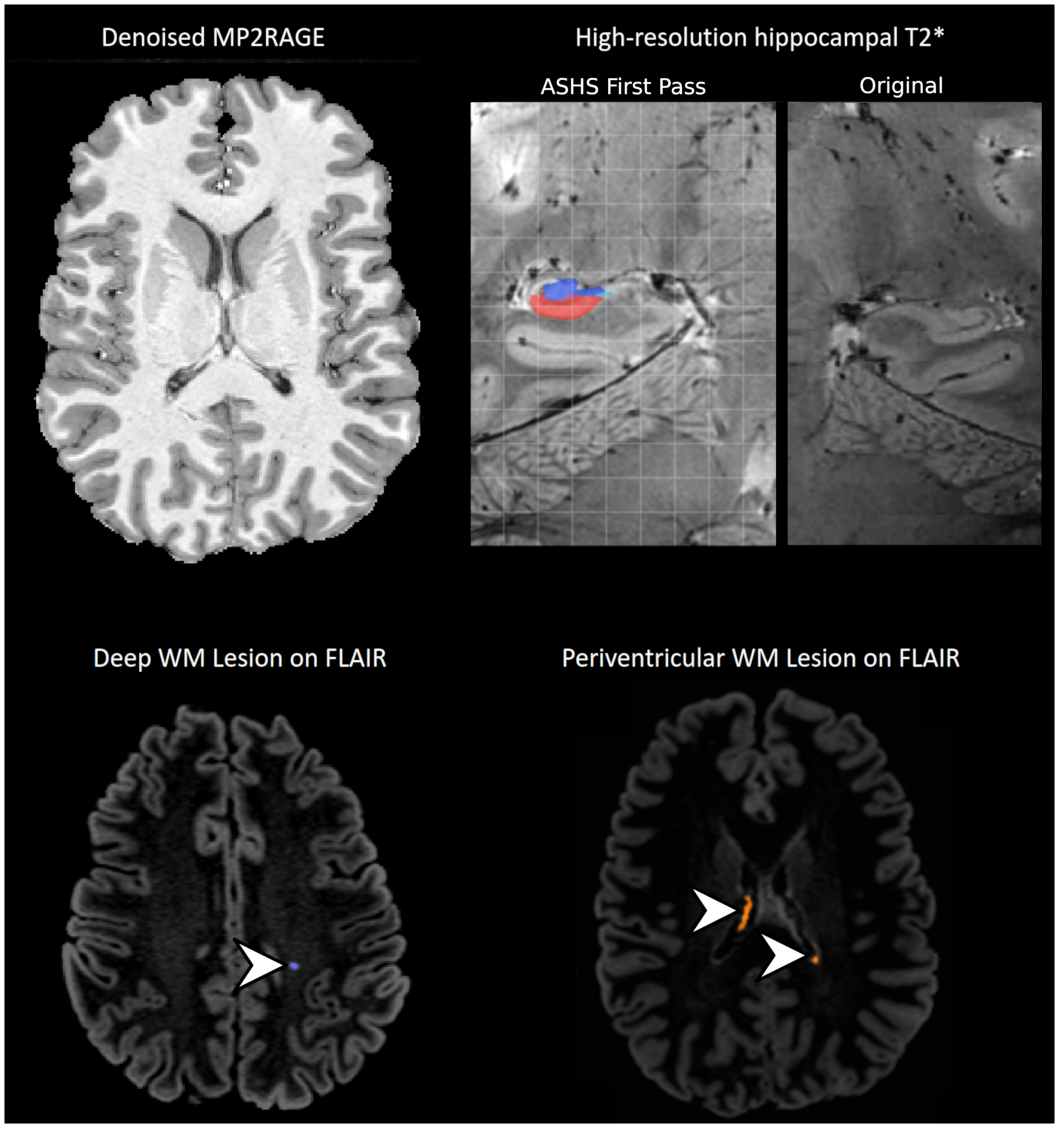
Example anatomical data from one participant in CUPS. Arrows point to lesions detected by Lesion-Mapper-BIDS in the deep white matter and periventricular regions. MP2RAGE: A magnetization prepared 2 rapid acquisition gradient echoes, WM: White matter, FLAIR: Fluid-attenuated inversion recovery.
